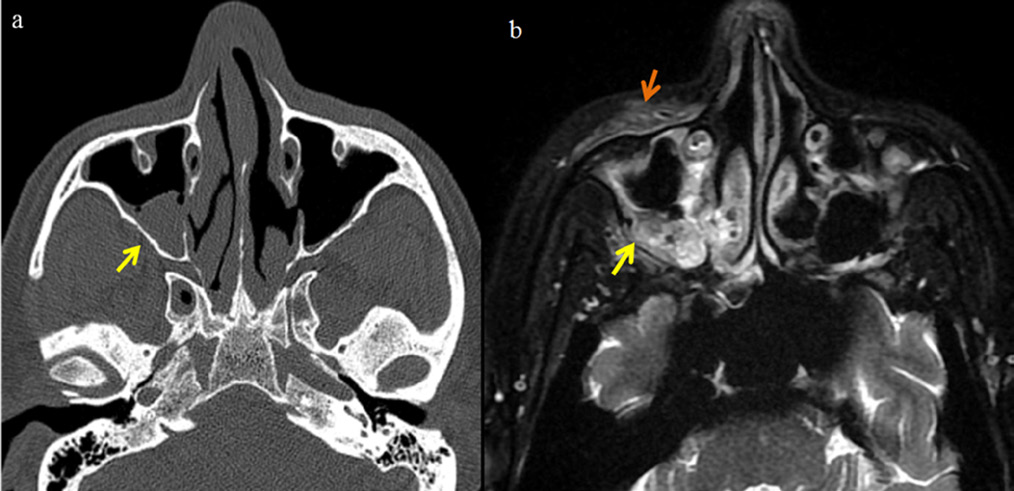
Axial CT bone window image (a) shows mucosal thickening in right maxillary sinus without any bone erosions (arrow). T2FS sequence in axial plane showing mucormycosis with soft tissue infiltration. Hyperintensity noted in right premaxillary soft tissues (orange arrow) and pterygopalatine fossa (yellow arrow).
